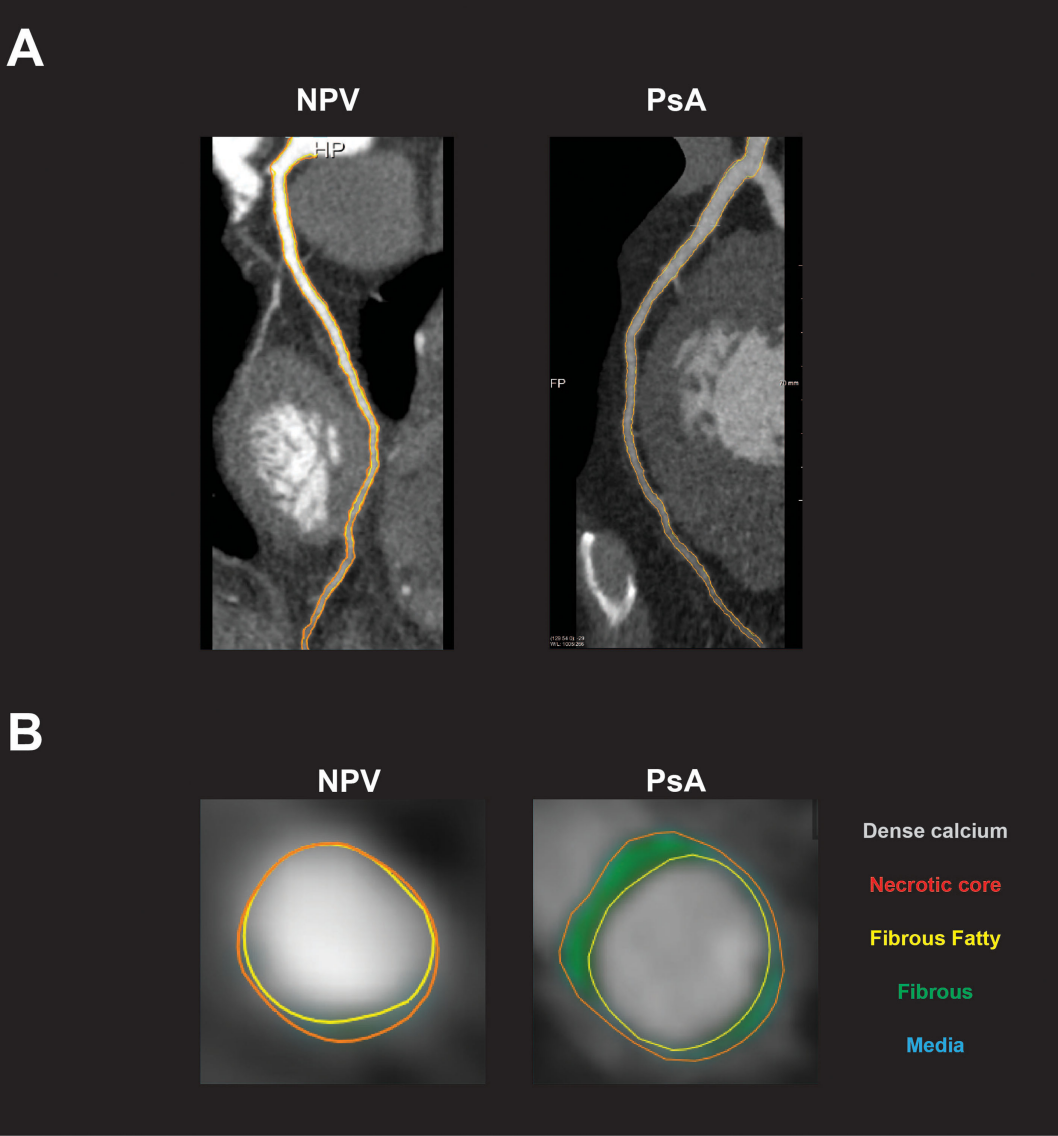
Subclinical coronary artery disease in patients with psoriatic arthritis. (A) Representative coronary artery CT angiogram (CCTA) images show left anterior descending (LAD) artery in non-psoriatic volunteers (NPV) and patients with psoriatic arthritis (PsA). (B) Representative CCTA images show coronary artery plaque in NPV and PsA. Total burden is determined by measuring the total plaque area (orange) around the lumen (yellow). Non-calcified coronary artery burden (NCB) is determined by calculating the difference between total and dense calcified burden. For these subjects, NCB x 100 = 0.602 (NPV) and 2.108 (PsA).
